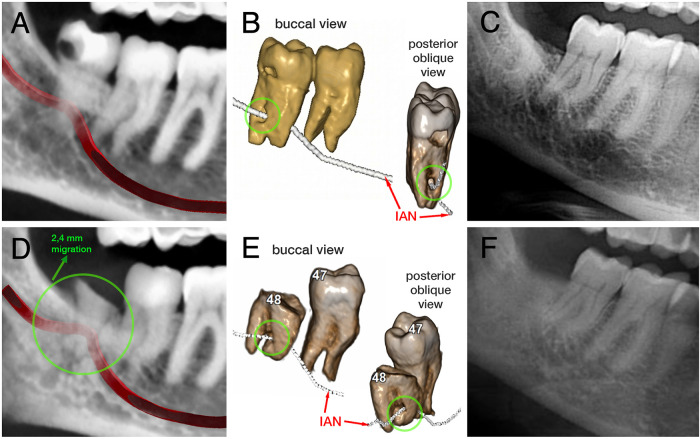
(A) Pre-operative reformatted panoramic image derived from CBCT data of right mandibular region, showing the mandibular canal trajectory (red). (B) CBCT-based segmentation demonstrating the relationship between the right M3, second molar and the inferior alveolar nerve (IAN); red arrow indicates the IAN course and green circles indicate the intimate root-IAN relationships; buccal view on the left and posterior oblique view on the right. (C) Immediate post-operative orthopantomography (OPG) after coronectomy of the right M3. (D) Two-year post-operative reformatted panoramic image reconstructed from CBCT data, the green circle and arrow demonstrate an estimated 2.4 mm of coronal migration of the retained roots and coronal displacement of the mandibular canal trajectory, consistent with presumed mechanical stretching of the IAN by the migrating root apices. (E) Two-year post-operative CBCT-based segmentation demonstrating the relationship between the right M3, second molar, and the inferior alveolar nerve (IAN); red arrow indicates IAN course and green circles indicate the intimate root-IAN relationship; buccal view on the left and posterior oblique view on the right. (F) One-year post-operative OPG, after secondary surgical removal of the retained roots, showing complete removal of the retained roots and absence of residual pathology.
